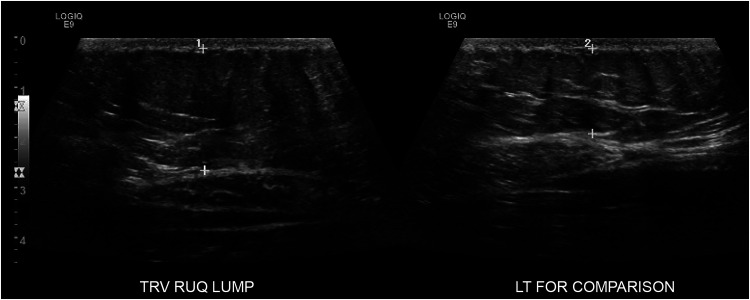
Ultrasound demonstrating region of abundant lipomatous tissue in the right upper quadrant with the left for comparison. TRV, transverse view; RUQ, right upper quadrant; LT, left.
